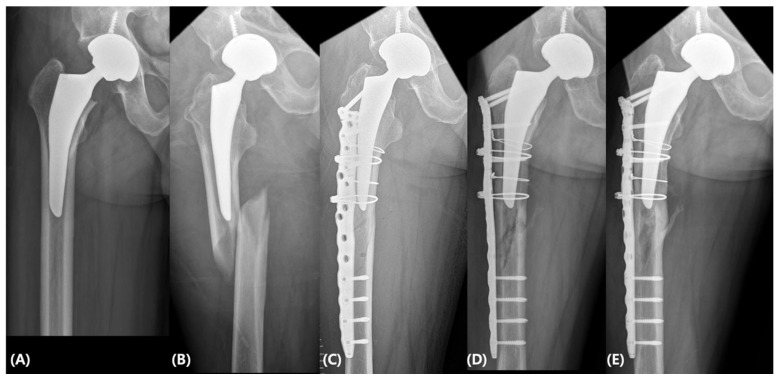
(A) A 60-year-old man who had undergone total hip arthroplasty for osteonecrosis of the femoral head 2 years earlier. (B) Radiographs obtained after a fall at 2 years postoperatively demonstrate a Vancouver type B1 periprosthetic femoral fracture. (C) Open reduction and internal fixation were performed using a locking plate and screws. (D) Radiographs at 3 months postoperatively demonstrate callus formation. (E) Radiographs obtained 8 years after surgery showing maintained firm fixation without evidence of implant failure.
